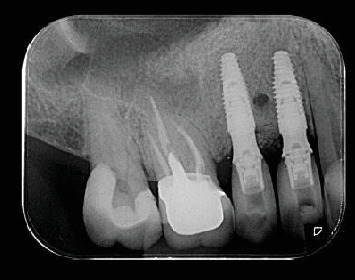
Periapical radiography of the implants placed with zirconia prosthesis rehabilitation.
